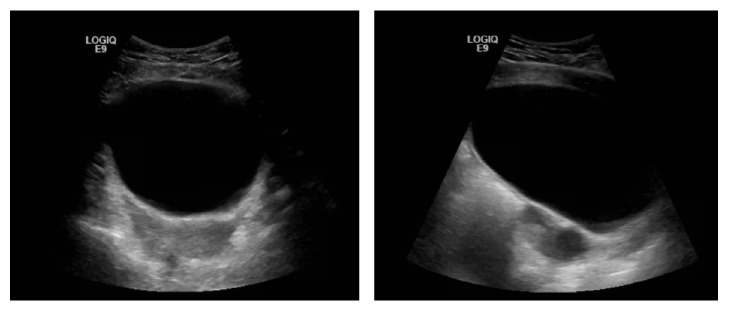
Abdominal ultrasound performed prior to cyst aspiration demonstrating recurrent, simple paravaginal cyst.
